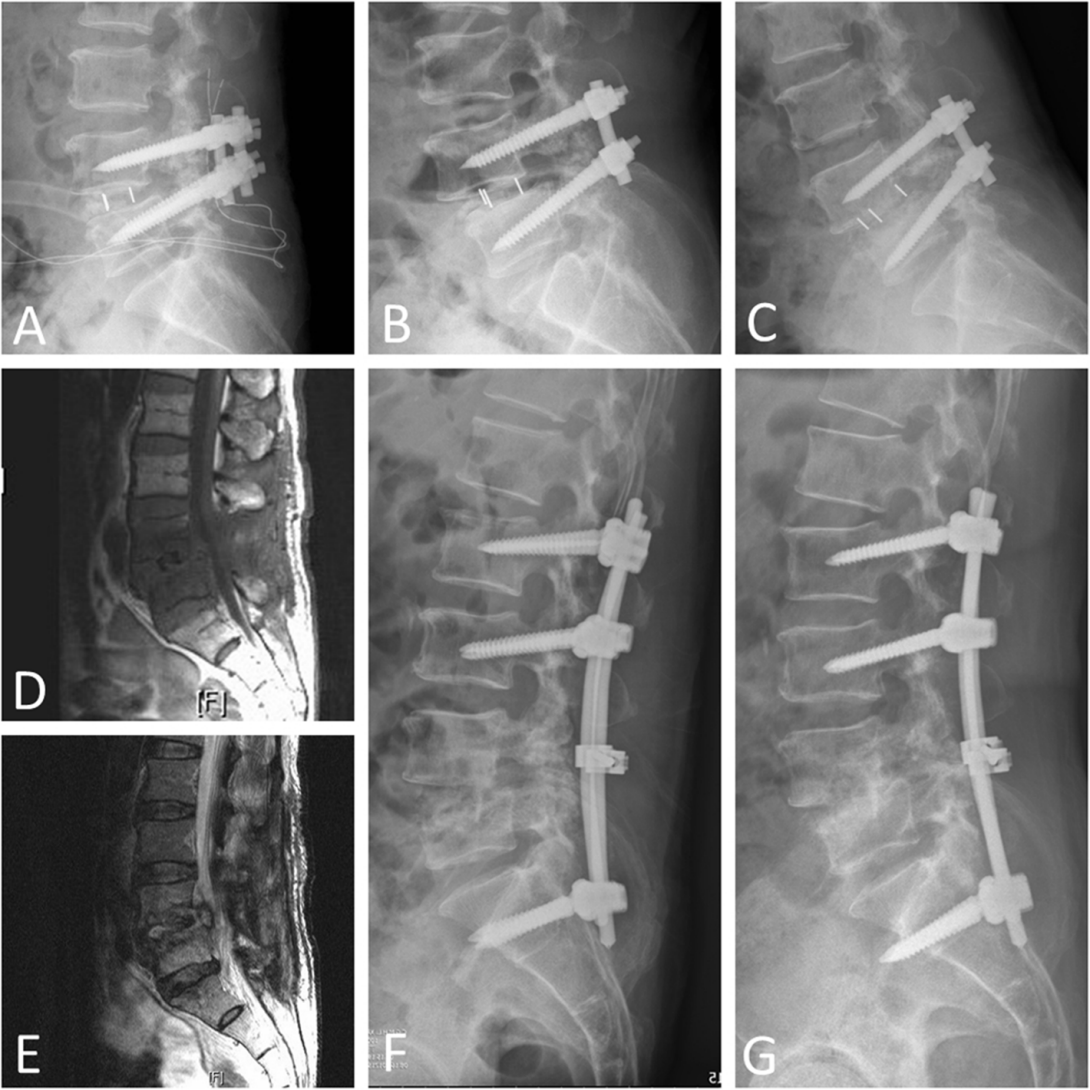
A A 70-year-old man underwent L4–L5 fusion with screws and cage. An initial postoperative X-ray showed good locations of the implants. B One month after the surgery, a follow-up X-ray showed posterior migration of the L4–L5 cage and suspected L5 screw loosening, and conservative treatment with oral analgesics and brace protection was chosen. C Three months after the surgery, progressive posterior migration of the L4–L5 cage, erosion of the L4–L5 end plates, and L5 screw loosening were noted. D, E T1-weighted and T2-weighted magnetic resonance imaging revealed low signal changes in the abutting L4 and L5 vertebrae, and fluid accumulation in the L4–L5 disc space, respectively. F An anterior + posterior approach was chosen. Posterior surgery for the upper and lower extension of the instrumented level was performed, followed by anterior surgery with cage removal and interbody fusion with autologous iliac tricortical bone graft. An initial postoperative X-ray showed good alignment. G Fourteen months after the anterior + posterior surgery, an X-ray revealed L4–L5 solid interbody union. The patient also had improved clinical outcomes and adequate infection control.
